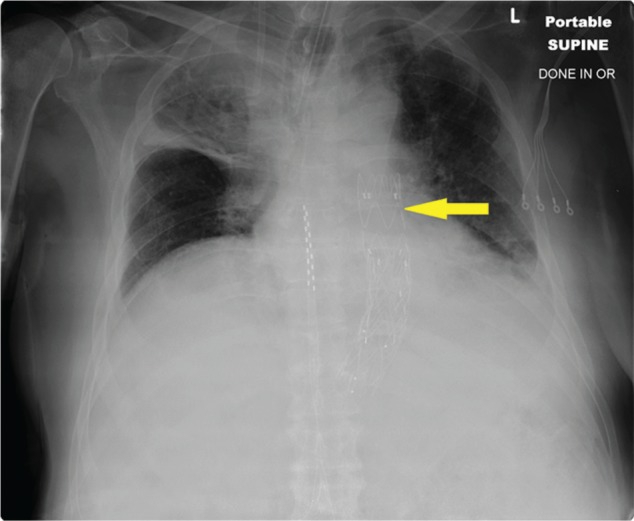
Follow-up chest X-ray after repair of aorta with transthoracic endovascular stent graft.
